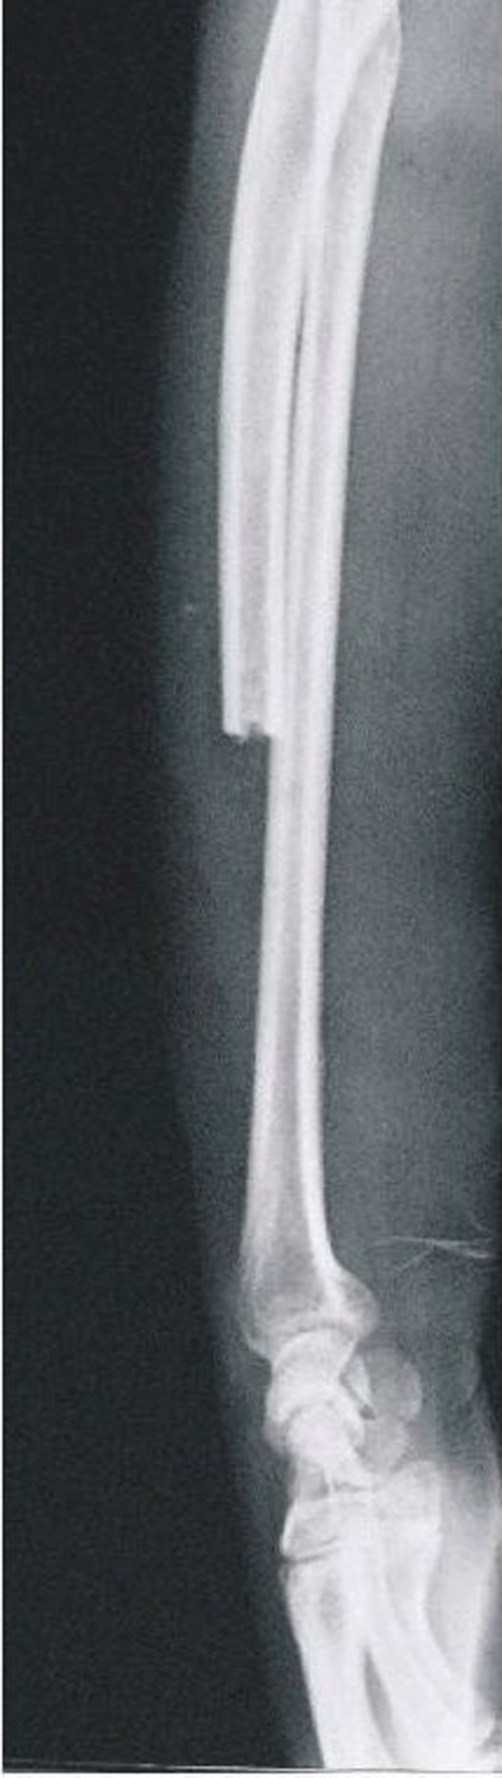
Lateral radiograph of Case #1 at 3 years of follow up.
